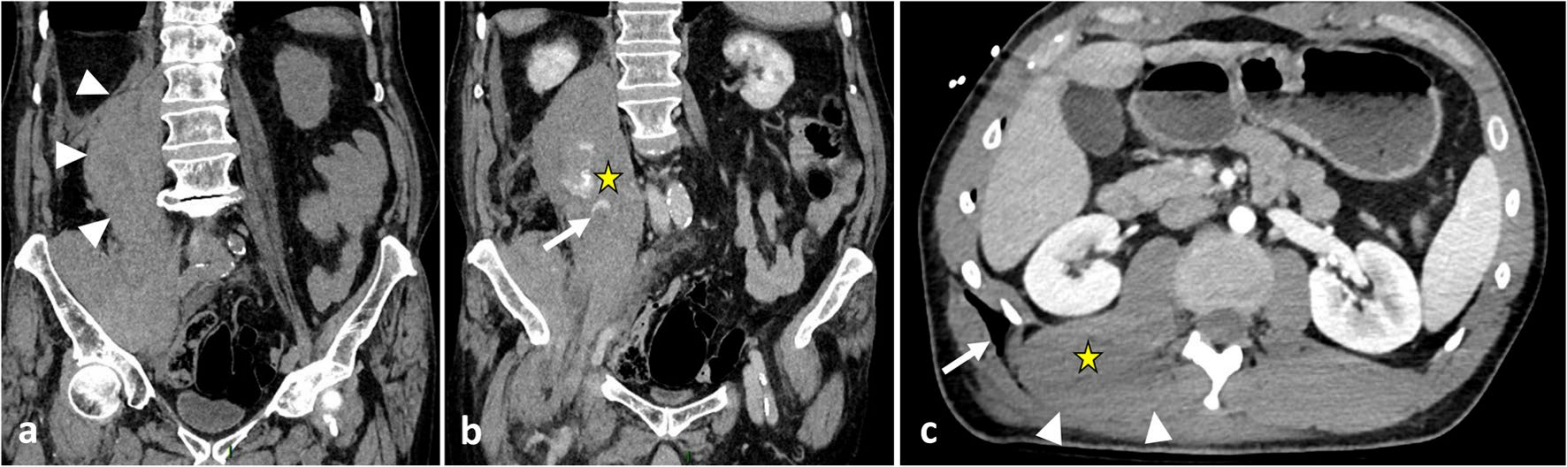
Trauma conditions* examples in in the paraspinal region. Right *psoas haematoma* in a 76-year-old female following a fall. Coronal CT scan reconstruction without (a) and with contrast agent (b) shows enlarged right psoas (arrowheads) with active extravasation of contrast (arrow). Muscle *contusion of the right erector spinae muscle* in a 27-year-old male after bicycle accident (c). Contrast-enhanced axial CT scan reconstruction (c) demonstrates enlarged erector spinae muscle (arrowheads) and subcutaneous emphysema (arrow). Yellow stars show epicentre of masses
